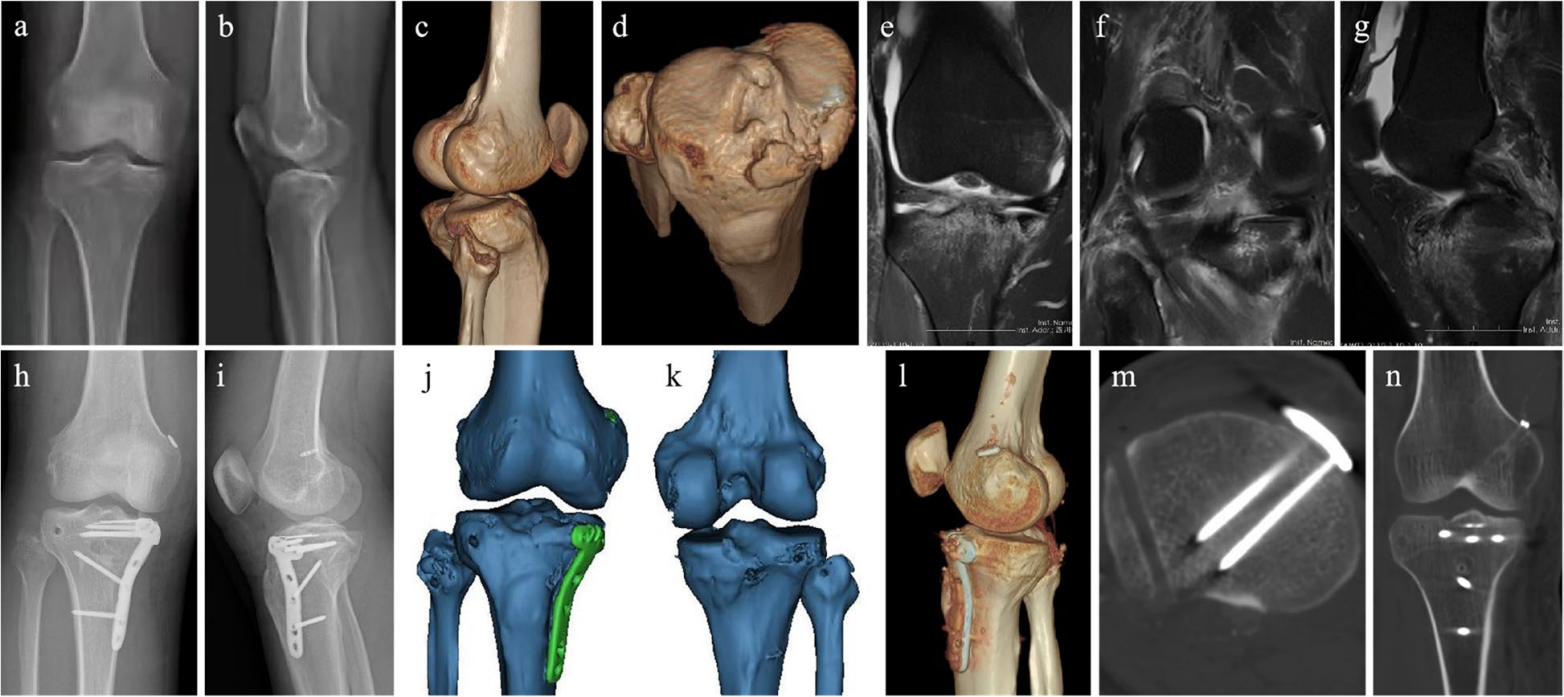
A 35-year-old man suffered a traffic accidence (case 2). a-d Preoperative X-ray and CT images showed the anteromedial tibial plateau and fibular head avulsion fracture. e–g Preoperative MRI images showed the fracture of the tibial plateau and injuries to PLC and PCL. h-n Postoperative X-ray and CT images showed that an anatomical reduction of the tibial plateau fracture, and the PCL and PLC tunnels were in a good position
